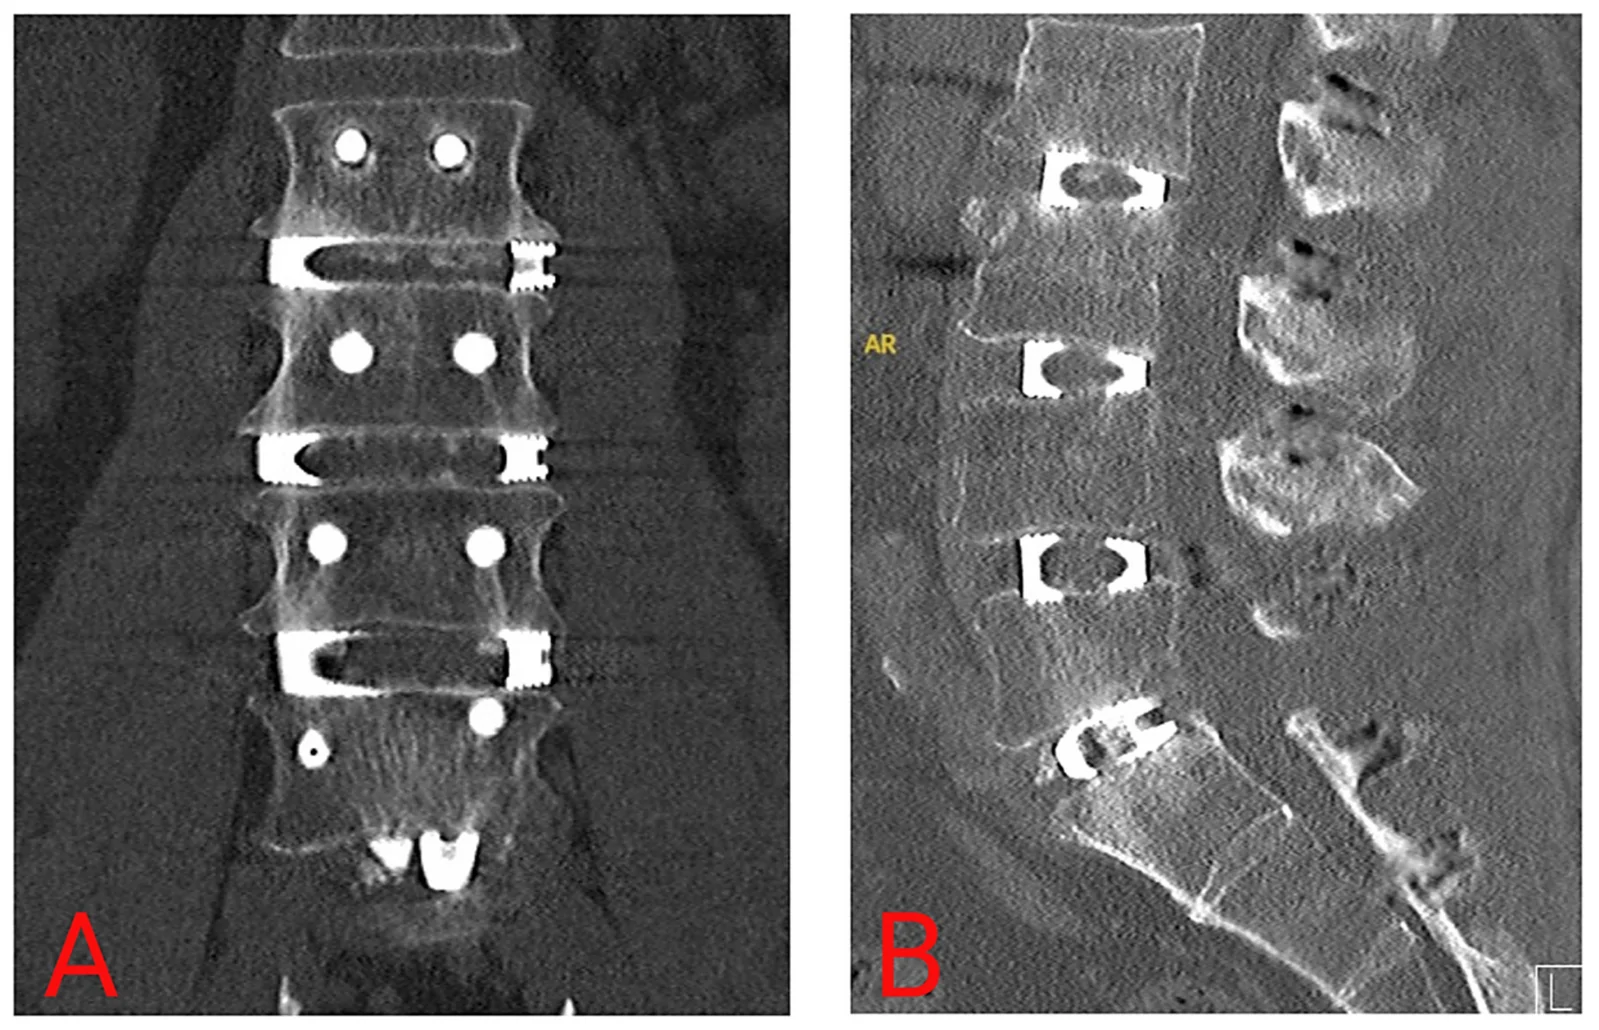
Coronal (A) and sagittal (B) computed tomography (CT) scans of the lumbar spine showcasing a multi-level posterior lumbar interbody fusion utilizing interbody fusion cages and pedicle screw instrumentation.
